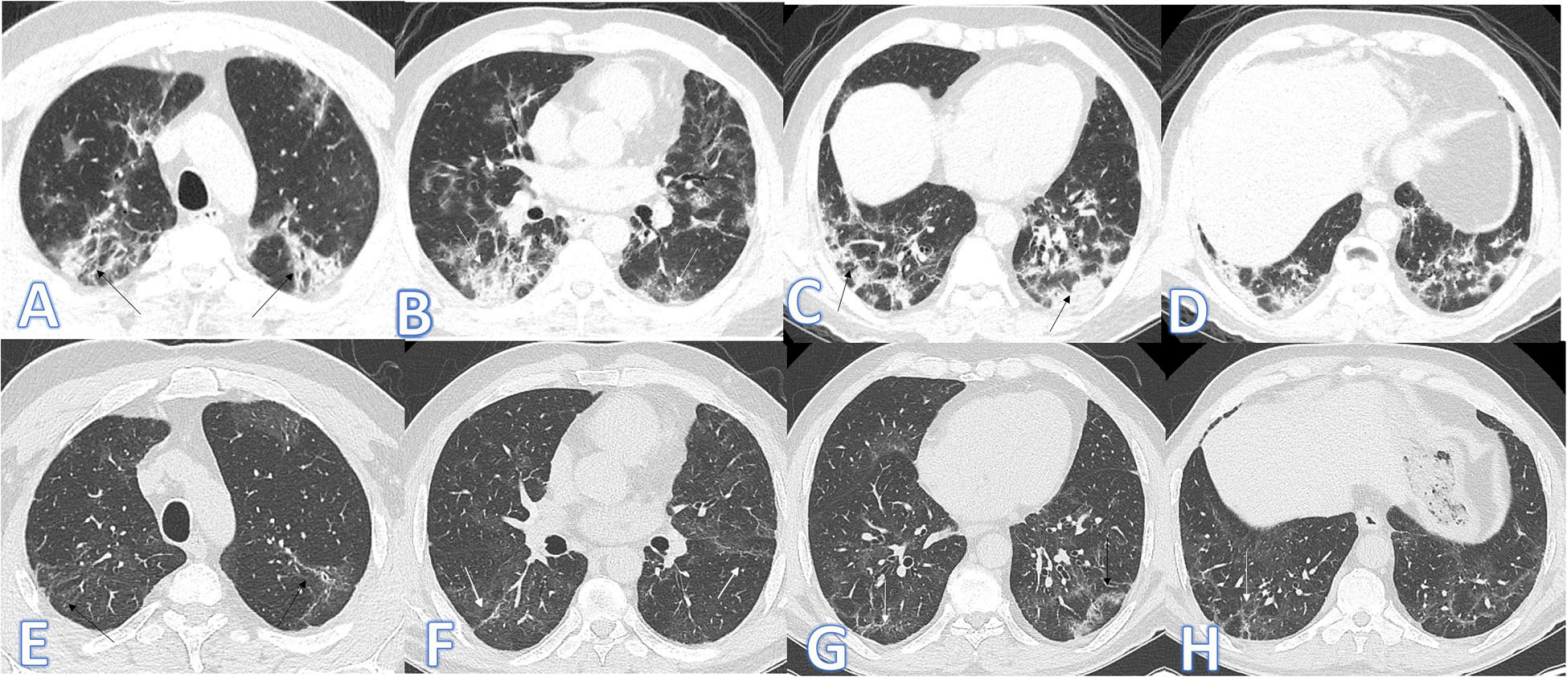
Fifty-nine-year-old male: he has DM; peak CT severity score 10; consolidation/crazy-paving score 8. No ICU admission; laboratory results show lymphopenia, high CRP, d-dimer, and serum ferritin. Steroid was given; length of stay during hospitalization is 10 days. Peak CT (a–d) shows bilateral peripheral consolidation patches mainly in the lower lobes (arrows). Follow-up CT 2 months after discharge (e–h) shows residual fibrosis with parenchymal bands, coarse reticular pattern (arrows), and atoll sign (black arrow in g).
